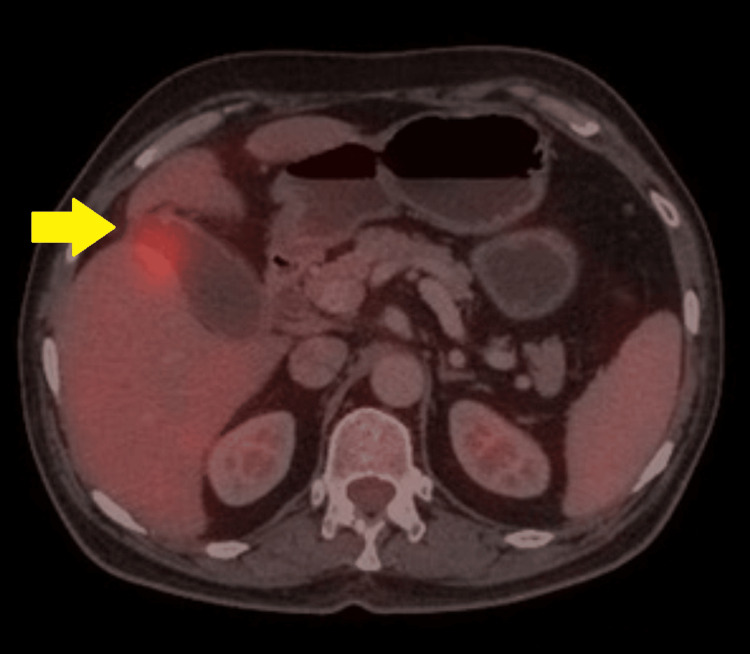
Preoperative FDG-PET CT (axial section) showing focal increased FDG uptake (yellow arrow) in the distal body and fundus of the gallbladder (SUV Max: 4.3). An eccentric thickening (around 7 mm) is seen in the fundus. FDG: Fluorodeoxyglucose F 18; PET CT: Positron Emission Tomography Computed Tomography; SUV: Standardized Uptake Value.
